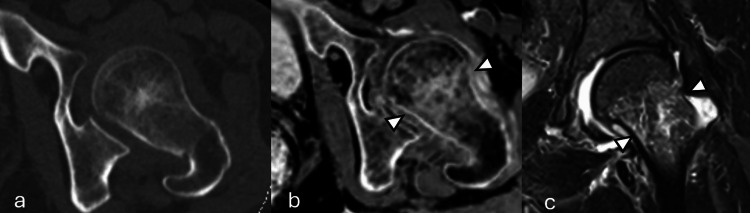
Representative imaging example 3. A patient with a non-displaced left subcapital femoral neck fracture (arrowheads) on (a) axial CT, (b) axial pseudo-CT, and (c) coronal short tau inversion recovery MR. The fracture was not visualized on CT but was conspicuous on pseudo-CT.
